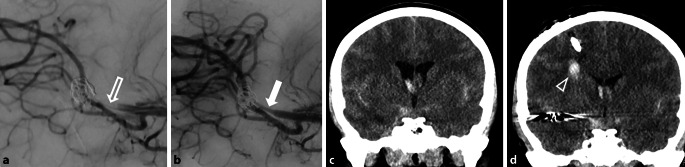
A patient with a ruptured middle cerebral artery (MCA) aneurysm treated with a flow diverter and coils. a After the procedure, in-stent thrombosis resulting in occlusion of an MCA branch (*open arrow*). b Recanalization of the branch (*closed arrow*) 20 min after initiation of tirofiban administration. c CT scan showing bilateral aSAH before EVD placement and endovascular treatment. d CT scan after EVD placement, intervention, and tirofiban administration, revealing an asymptomatic catheter tract hemorrhage (*arrowhead*) along the trajectory of a failed EVD placement attempt
